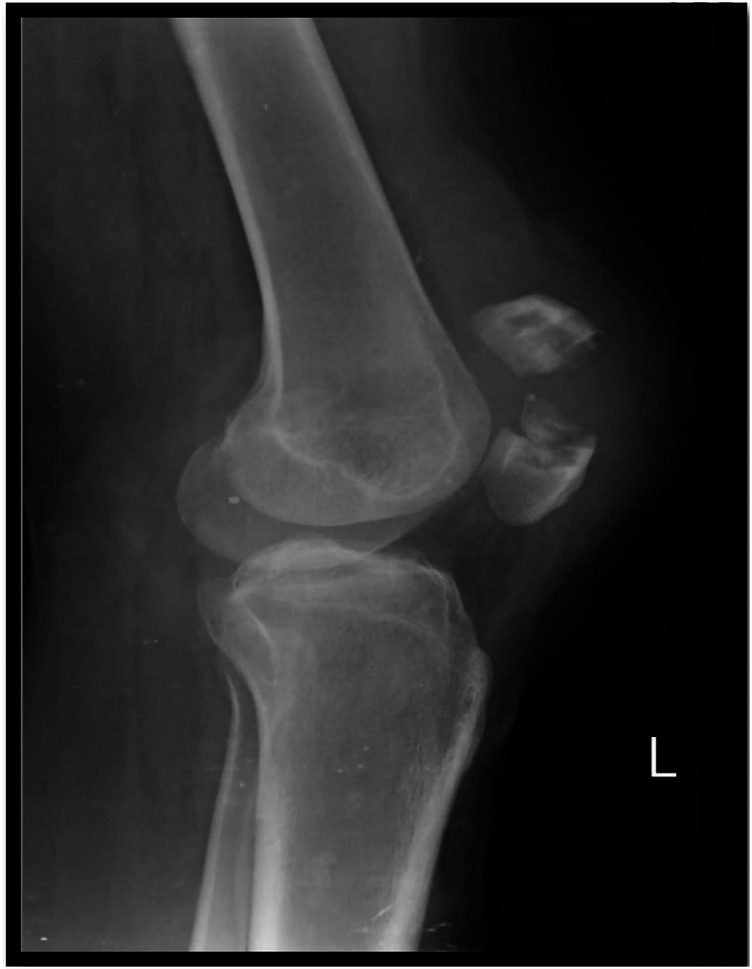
Shows the lateral x-ray of the left knee demonstrates a displaced transverse fracture of the patella, located at the mid-patellar level and there is displacement of fracture fragments, with the superior and inferior poles no longer in anatomical alignment.
